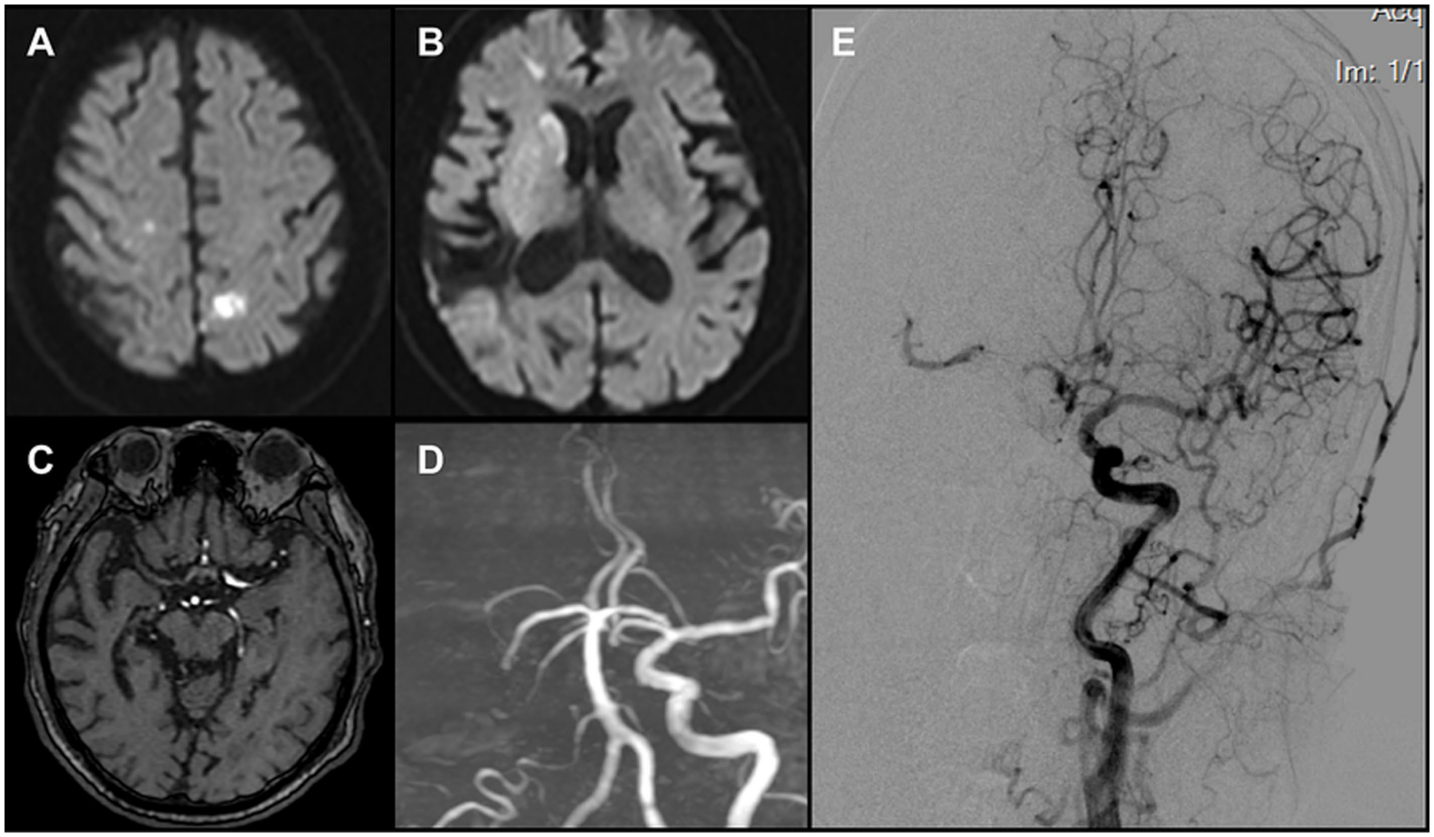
(A,B) DWI revealed multiple acute infarcts involving the right caudate head and the bilateral frontal and parietal cortices. (C) Original MRA images demonstrated non-visualization of the right middle cerebral artery (MCA). (D) TOF 3D reconstruction demonstrated non-visualization of the right internal carotid artery (ICA) and the right MCA (E) Left carotid angiography indicated a patent anterior communicating artery. A thrombus was identified in the proximal right MCA, causing subtotal occlusion, while compensatory flow to the MCA territory was provided by the collateral circulation.
